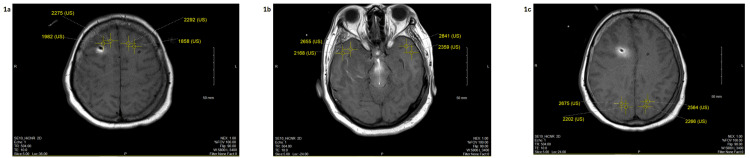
Patient 1 MRI intensities 12 hours post-IT contrast 1a: Frontal lobe; 1b: Temporal lobe; 1c: Parietal lobe IT: Intrathecal
